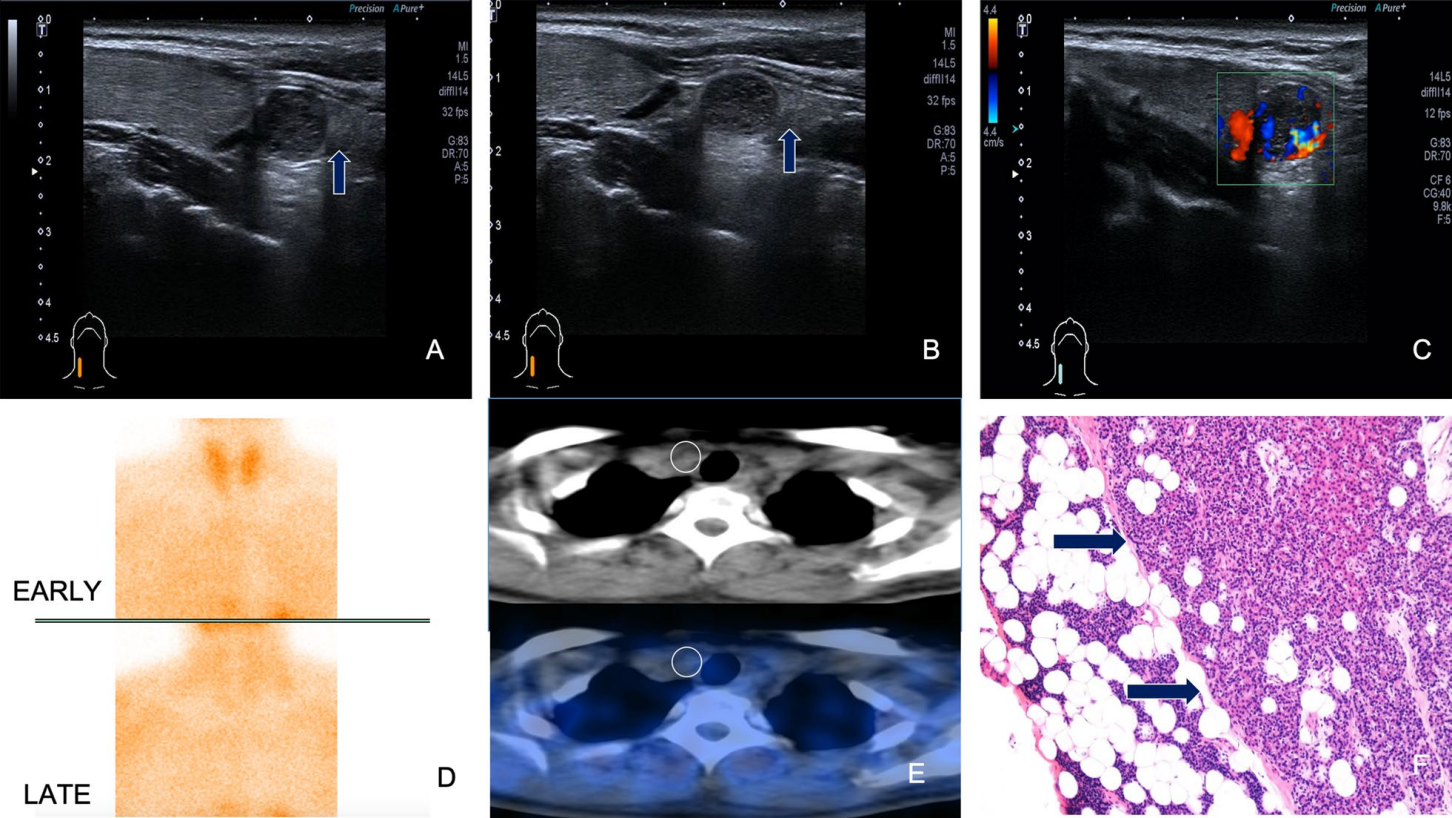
Parathyroid adenoma below the inferior pole of the right lobe of thyroid. The right inferior parathyroid adenoma clearly represents the residual parathyroid sign. Nodule and the thyroid gland move relatively with swallowing (a, b). Abundant blood flow signals on CDFI (c). The nodule was negative on planar images and Tc-99m MIBI SPECT/CT (d, e). The pathological results showed parathyroid adenoma (mainly chief cells and eosinophils), and fibrous capsule formation could be seen with the surrounding normal parathyroid gland (f)
